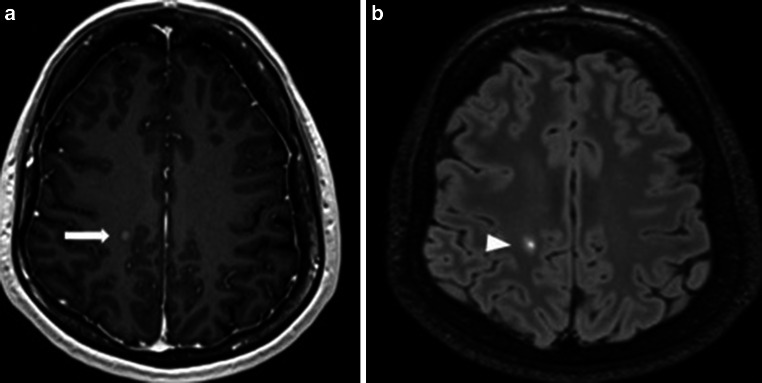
Active MS lesion (Gd+ lesion) in a primary progressive MS patient. a Axial T1-weighted image after contrast administration in a PPMS patient shows a gadolinium-enhancing lesion (*white arrow*) in juxtacortical white matter. b Corresponding section of axial fluid attenuated inversion recovery (FLAIR) image with hyperintense white matter lesion (*arrowhead*) in the same location
